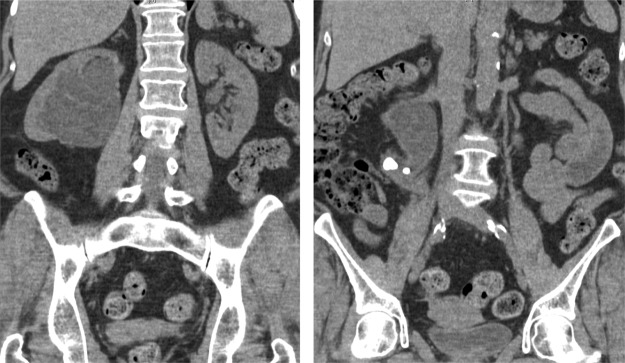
Preoperative computed tomography scan, coronal planes showing marked hydronephrosis associated with the presence of two calculi and in the ureteropelvic junction (UPJ) and an extremely tortuous ureter.
